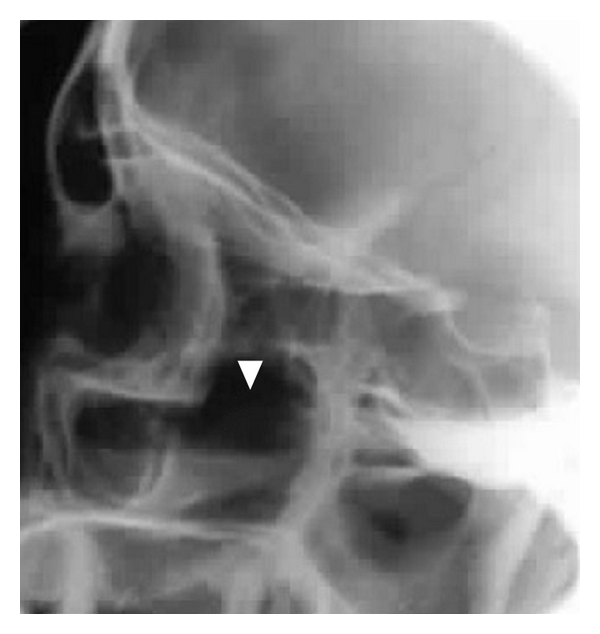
Lateral plain X-ray showing air-fluid level in the maxillary sinus.
